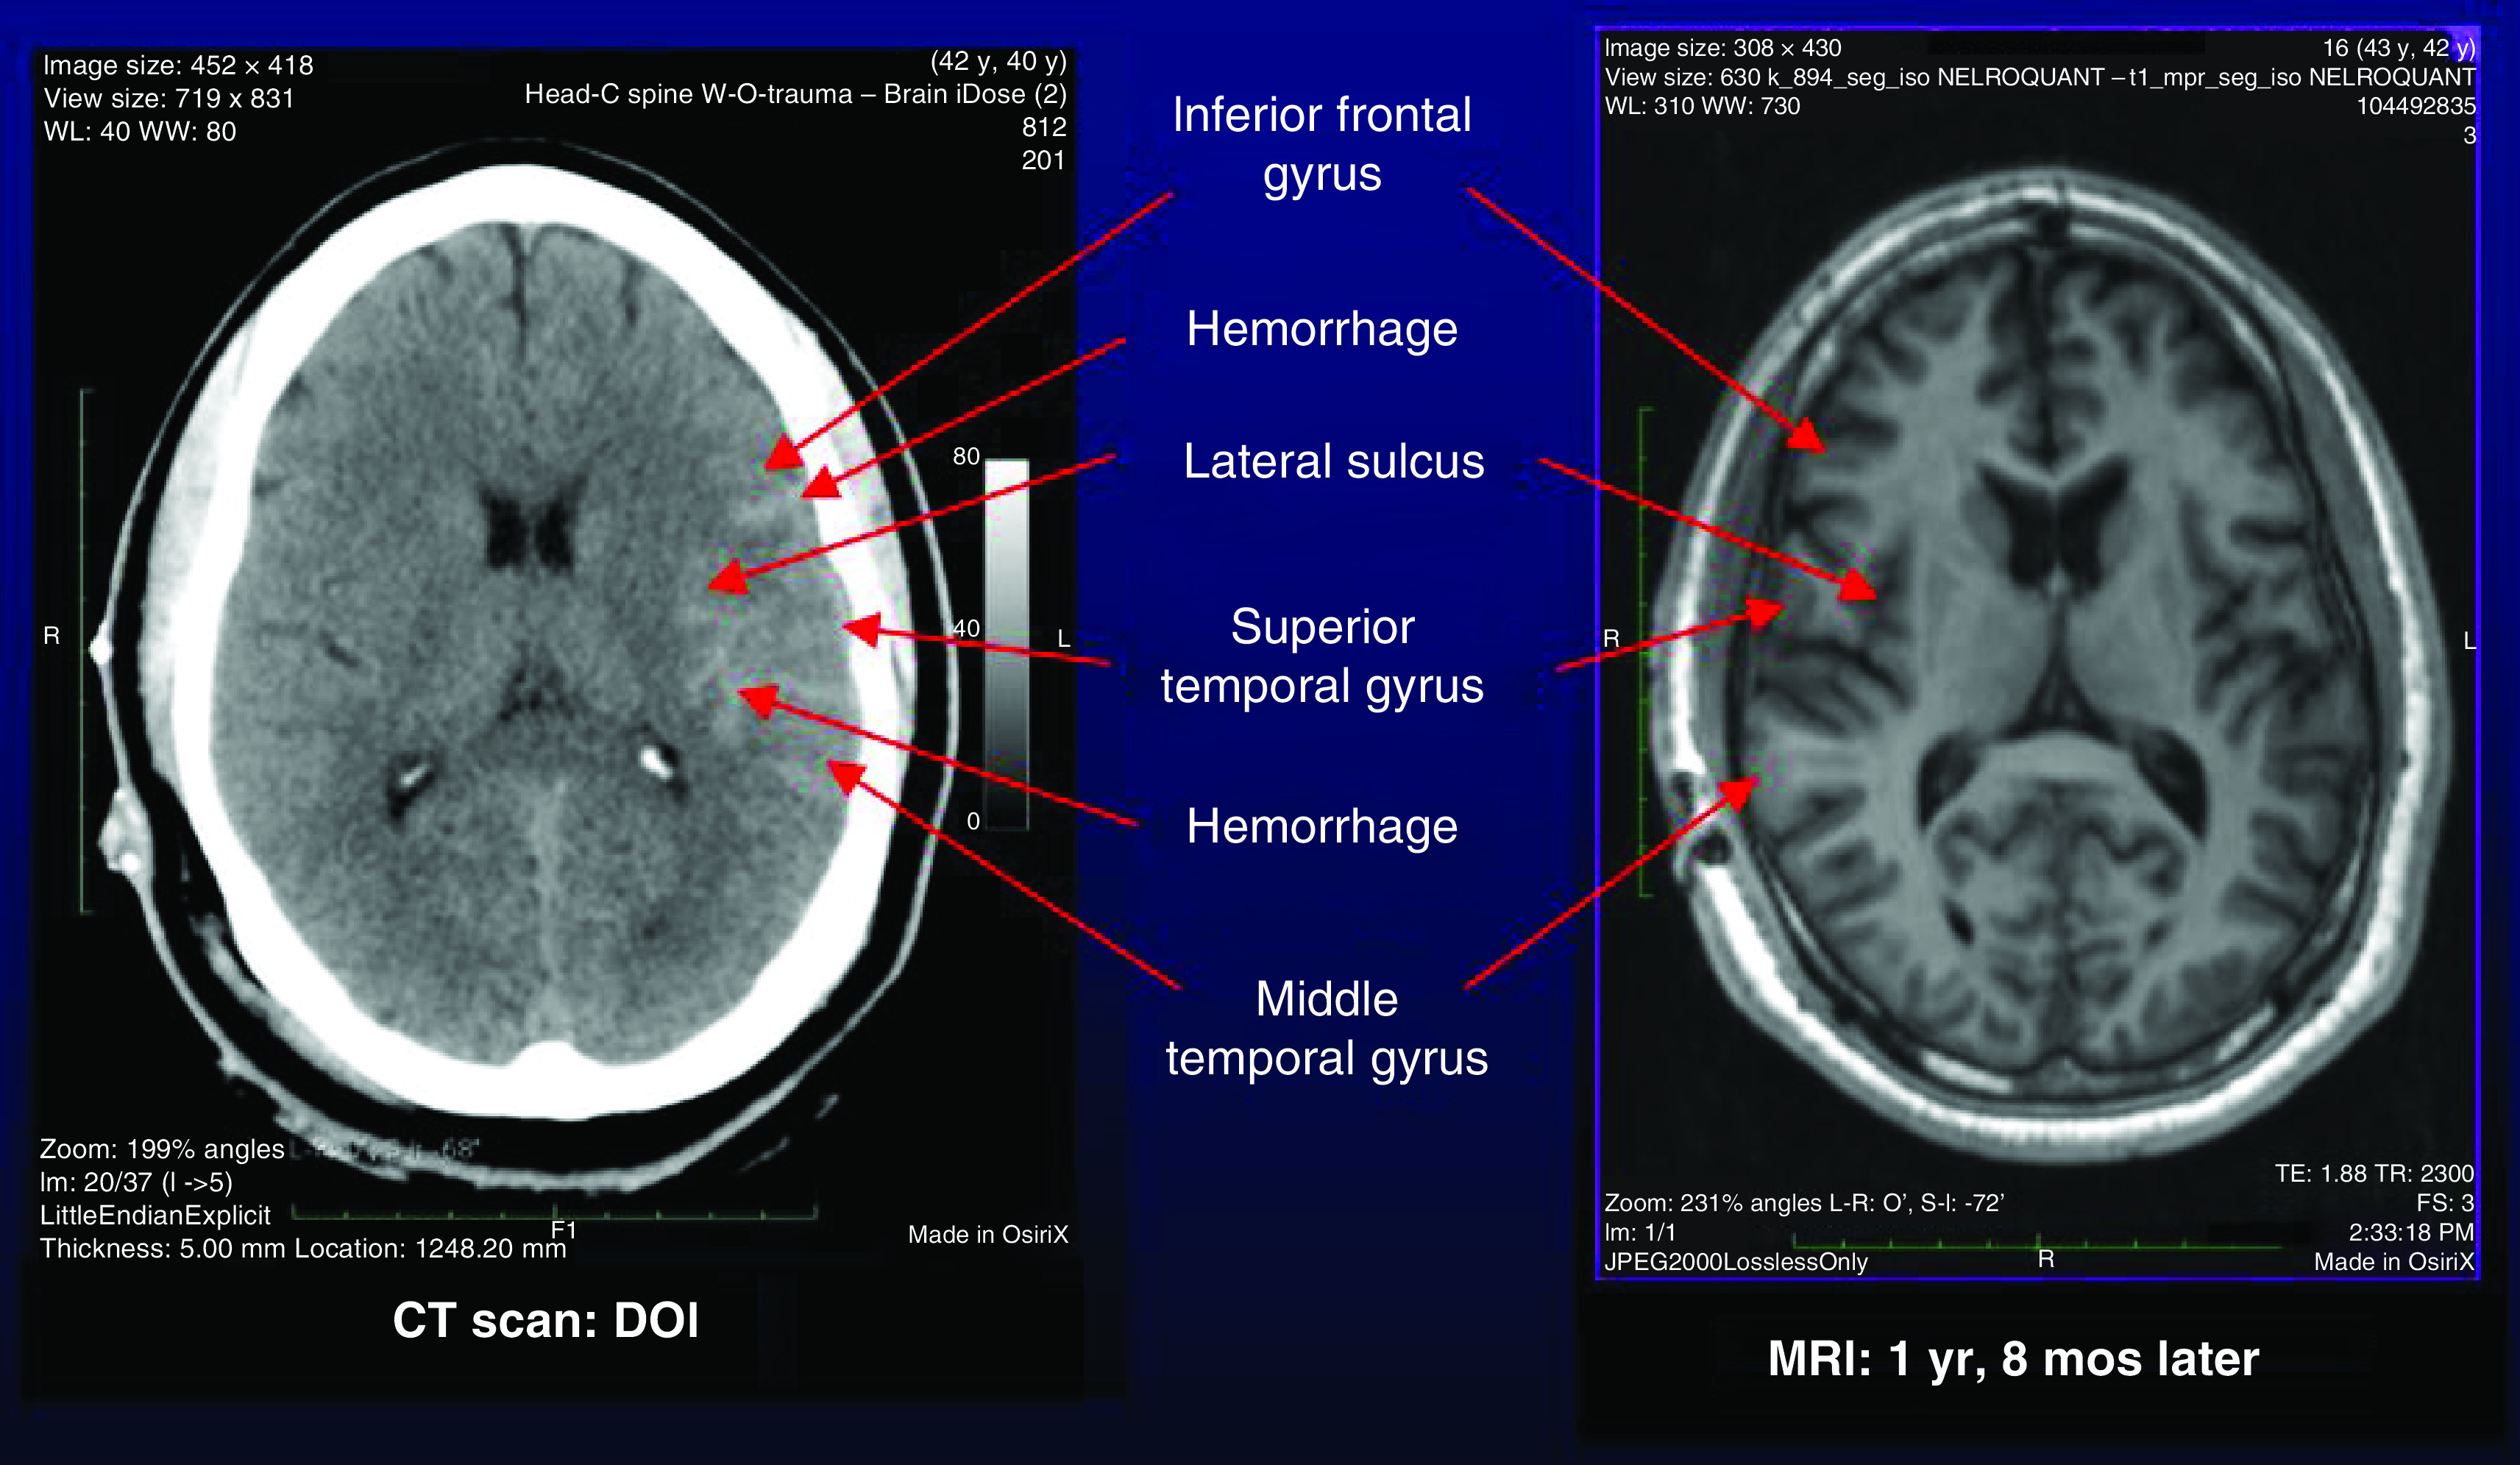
Patient RT had left cerebral hemorrhage on the day-of-injury CT scan, indicating greater forces to the left side of his brain. On follow-up MRI 1 year and 8 months later, he appeared to have normal brain structure. However, NeuroQuant^®^ and NeuroGage^®^ volume analyses showed left-sided cerebral atrophy, abnormal enlargement of many right-sided brain regions and abnormal asymmetry of many brain regions with R >L. These findings suggested that the right cerebral regions became enlarged due to compensatory hypertrophy.
